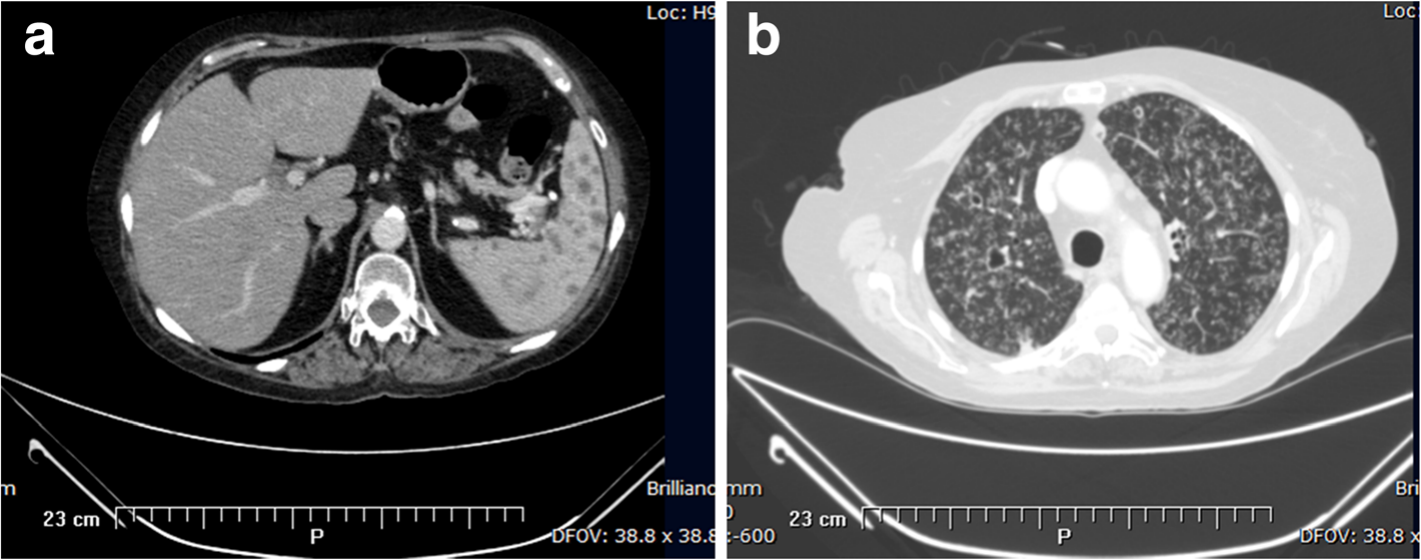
a Axial CT abdomen image demonstrates numerous hypo-attenuating lesions on spleen, almost replacing the normal parenchyma. b Axial CT chest image reveling reticulonodular infiltrates with tree in bud and cavitations
